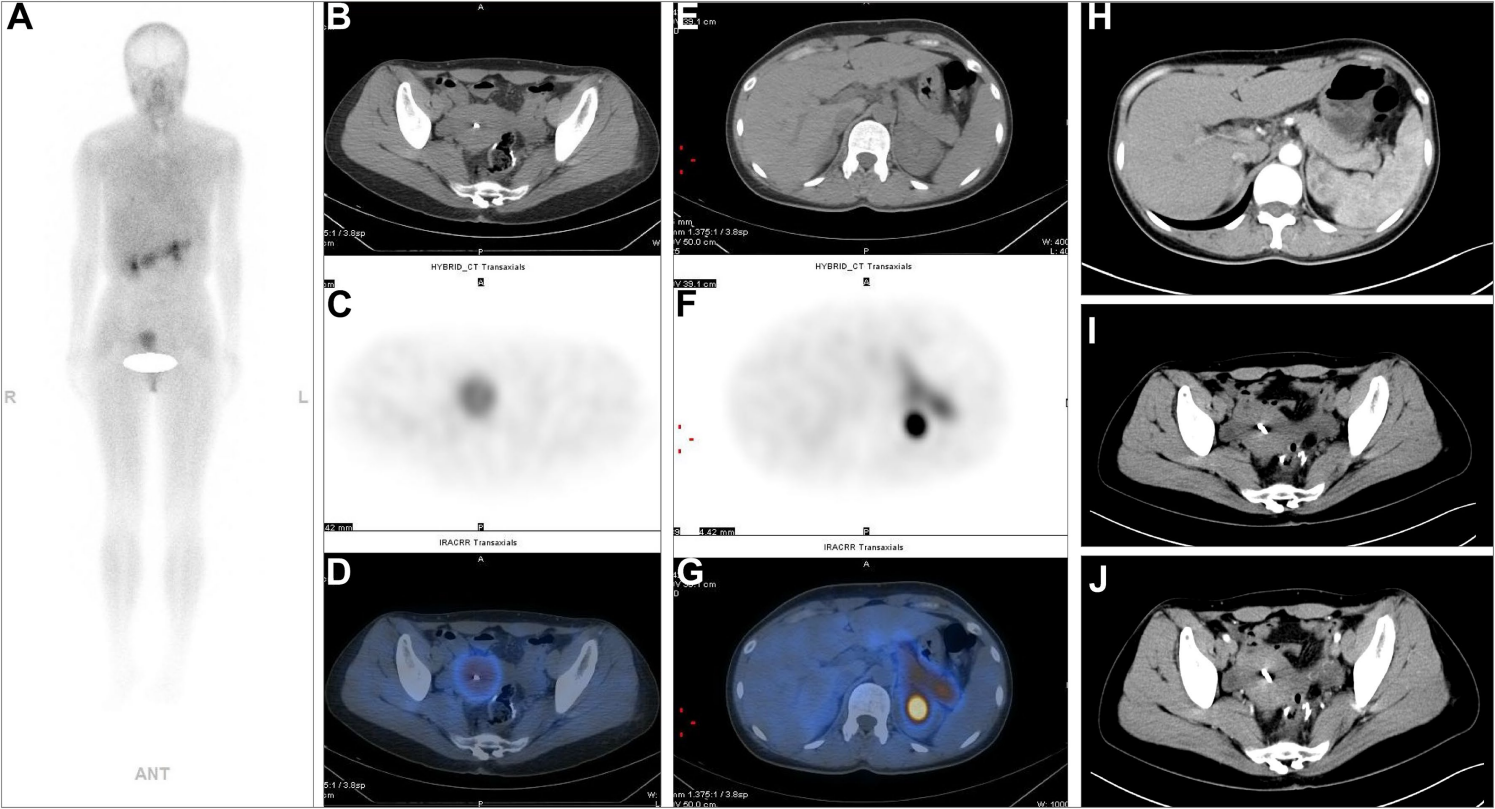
A 36-year-old postoperative female patient with sigmoid colon adenocarcinoma underwent ^99m^Tc-FAPI-04 SPECT/CT and CECT examinations. The whole-body maximum intensity projection (MIP) image (A). The axial CT image (B,E), axial SPECT image (C,F), and the corresponding fused ^99m^Tc-FAPI-04 SPECT/CT image (D,G). Metabolic foci with abnormal ^99m^Tc-FAPI-04 uptake were not detected on whole-body SPECT/CT. ^99m^Tc-FAPI-04 had high physiological uptake in the normal pancreas and uterus. Neither of the techniques showed thickening of the bowel wall at the anastomotic site, and enlarged lymph nodes were not detected in the abdominal or pelvic cavity via abdominal CECT (H–J).
